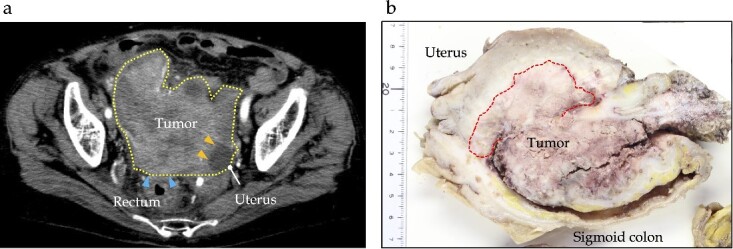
Preoperative and pathological findings of sigmoid carcinoma. (a) Contrast-enhanced computed tomography reveals a hypodensity mass (dotted line) of ~12 cm, located in the sigmoid colon, suspiciously invading the rectum (arrows) and uterus (yellow arrows). (b) Macroscopic findings reveal a type 1 tumor measuring 130 × 40 × 25 mm in the sigmoid colon, with direct invasion into the uterine corpus, left ovary, and small intestine.
